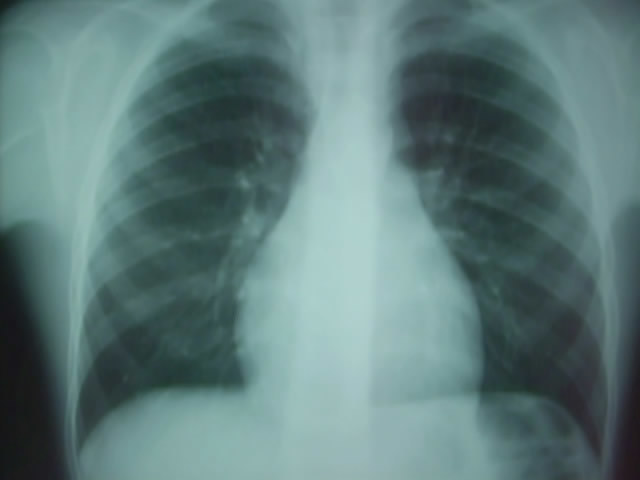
Radiographie du thorax montrant un arc moyen gauche en double contours
